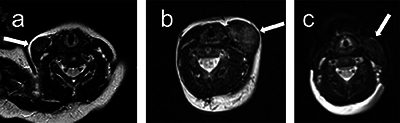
T2-weighted axial images from patients 1, 2 and 4 (labelled a–c) showing heterogeneous T2 hyperintensity of the fibromatosis colli (arrows).
